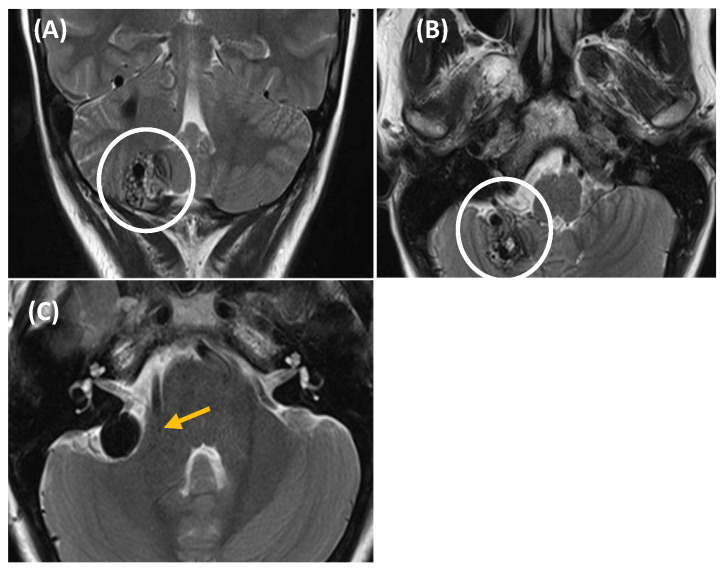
T2-weighted MRI showing a right cerebellar arteriovenous malformation (AVM) in a 44-year-old woman presented with somatosound, instability, and a sensation of pressure in the right hemiface. Coronal (image (A)) and axial (image (B,C)) MRI revealed a right cerebellar AVM, with a capillary nidus measuring 23 × 20 × 19 mm (outlined by a white circle in images (A,B)). The AVM showed multiple draining veins towards the anterior petrosal sinus and voluminous varicose venous dilatation (yellow arrow in image (C)) adjacent to the posterior border of the IAC. Given the size, location, and characteristics of the AVM, the decision was made to treat the patient using radiosurgery with Gamma Knife. This technique targets the abnormal vessels, promoting gradual occlusion of the AVM while minimizing damage to surrounding brain tissue.
